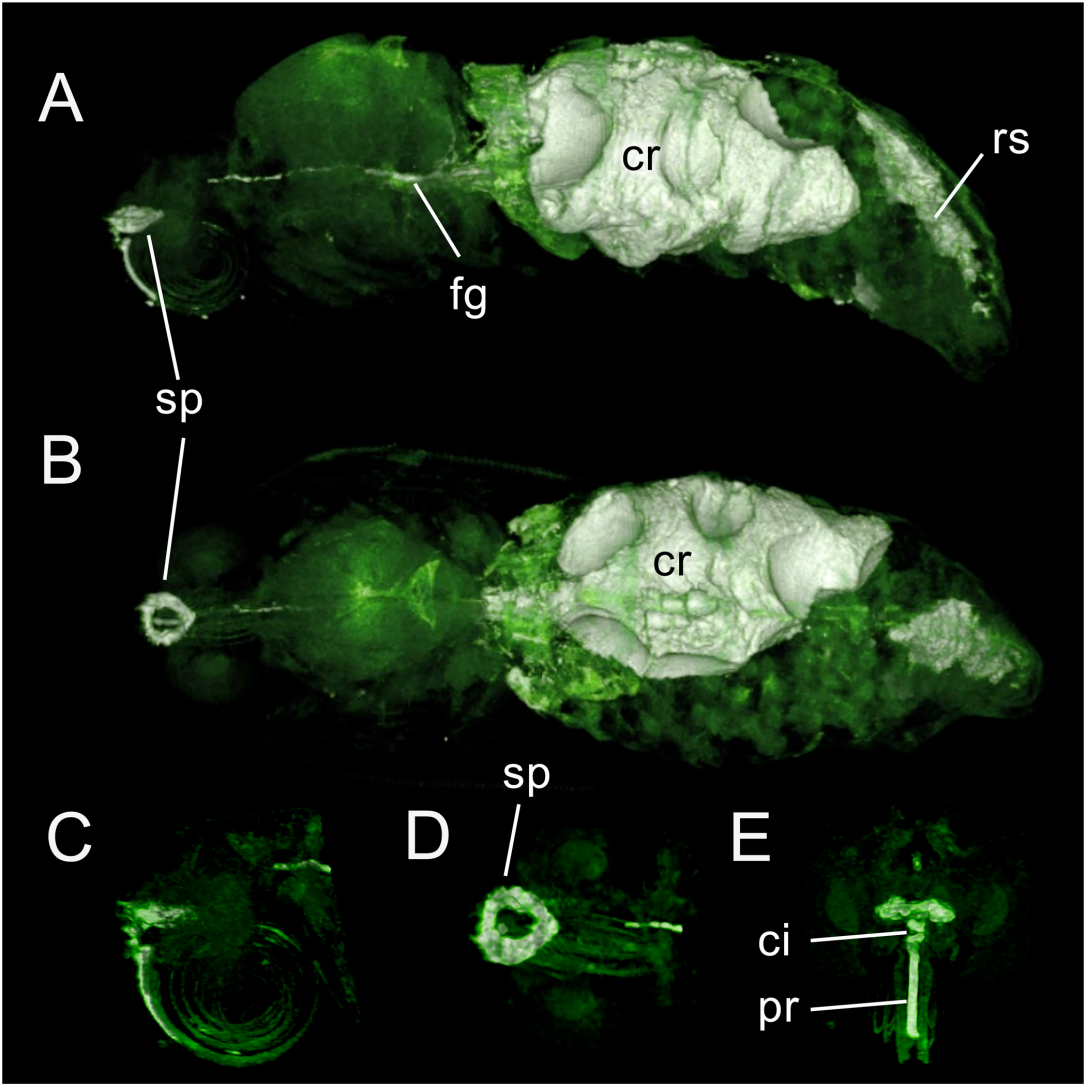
3D-GRE MR imaging data acquired from a *M*. *sexta* adult female. (A) Dorsal 3D surface-rendered view. Air sacs and ovarioles induce irregularities in the crop (cr) surface. Proboscis (pr), cibarium (ci), sucking pump (sp) and foregut (fg) are visible; the midgut is hidden in this view. The rectal sac (rs) is now deflated. (B) Coronal view showing the sucking pump, foregut and crop. The anterior portion of the midgut lies immediately anterior to the crop. Darkened circular structures in the abdomen are eggs. (C) Mid-sagittal view of the proboscis, cibarium, sucking pump and foregut. The cibarium lies just ventral to the sucking pump. (D) Dorsal view of the sucking pump. (E) Frontal view of the proboscis, cibarium and sucking pump.
